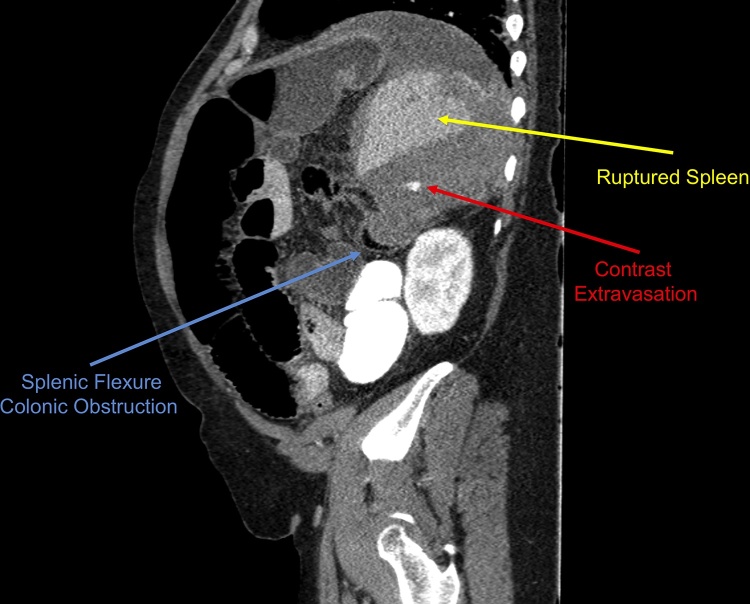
Post-traumatic splenic hematoma with active contrast extravasation causes extrinsic compression of splenic flexure, resulting in large bowel obstruction. Computed tomography coronal sagittal section on initial presentation.
